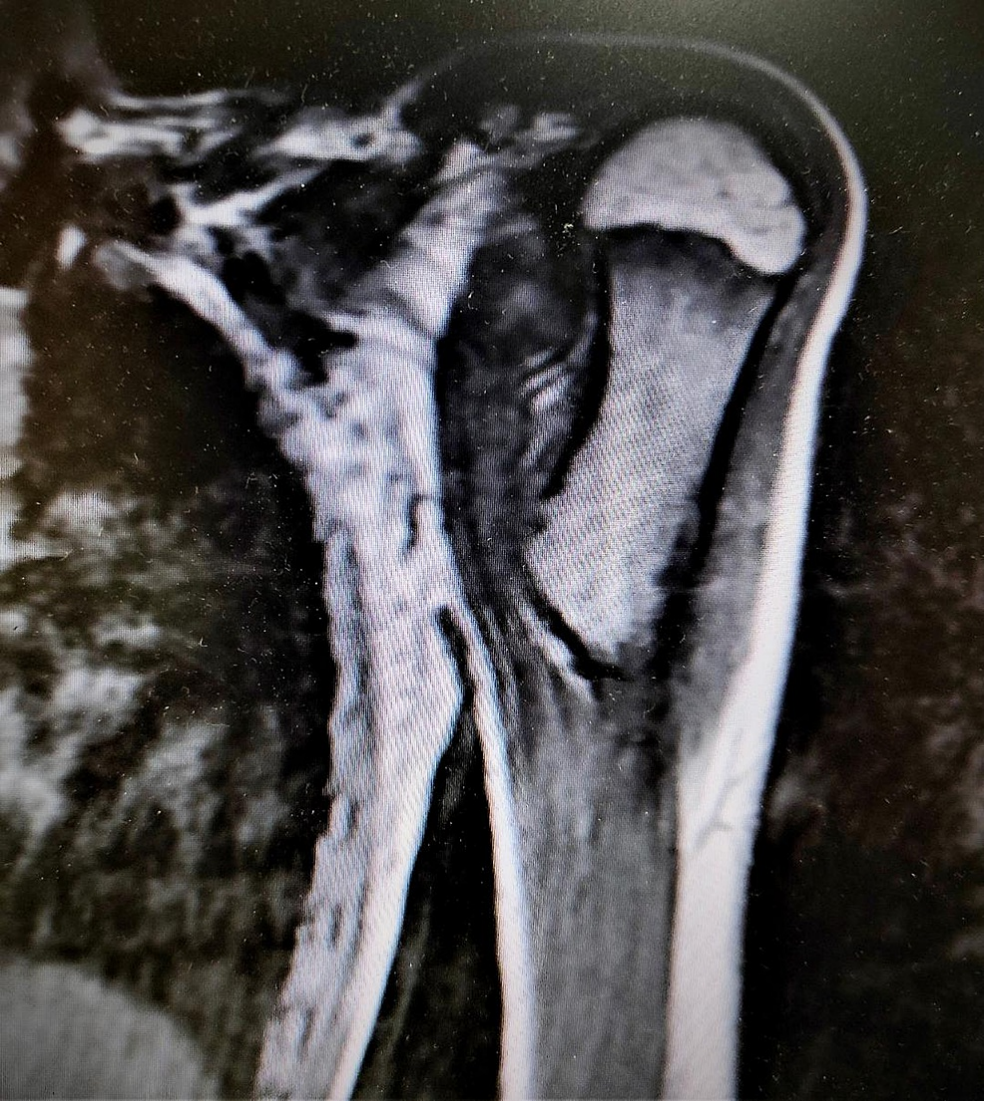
Left shoulder MRI showing a 4-cm bony protuberance covered by a 4-mm cartilage cap (osteochondroma).
